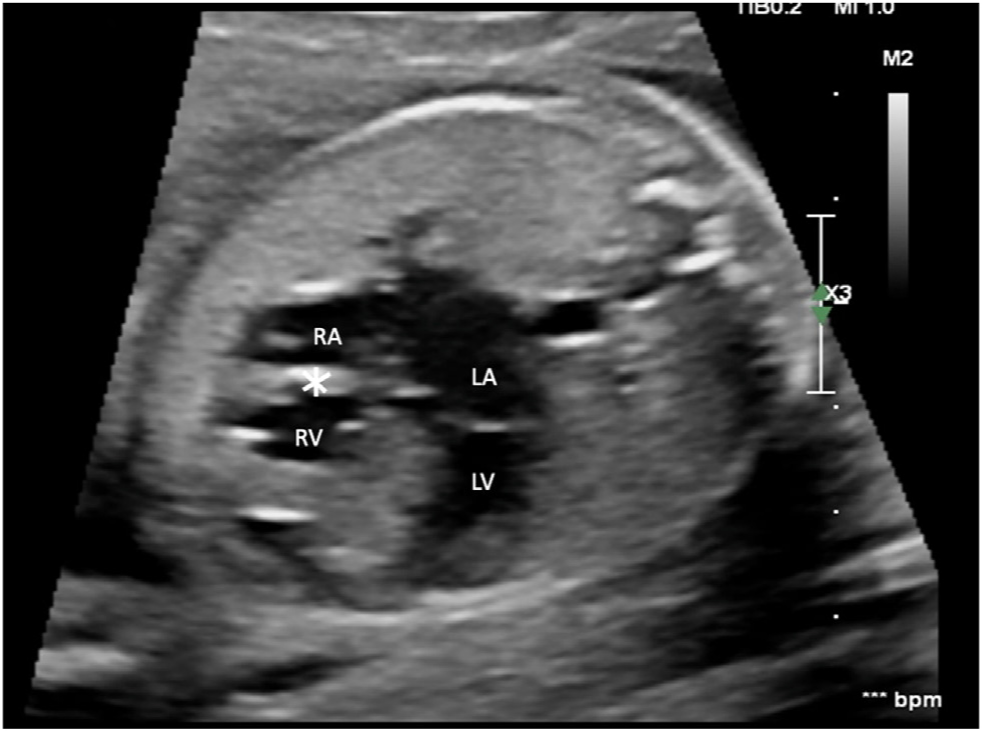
Transabdominal two-dimensional fetal echocardiogram, 4-chamber view, demonstrates a severely hypoplastic, hypertrophied, and aneurysmal RV with an atretic tricuspid valve (*asterisk*). *LA*, Left atrium; *RA*, right atrium.
